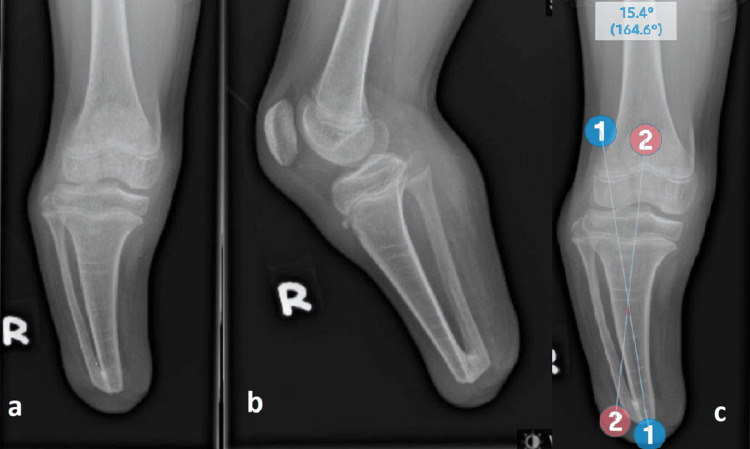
Plain radiograph in (a) anteroposterior and (b) lateral views of the knee joint showing varus deformity of the tibia, proximal migration of the fibula and distal tibiofibular synostosis. (c) Preoperative measurement of the angle.
